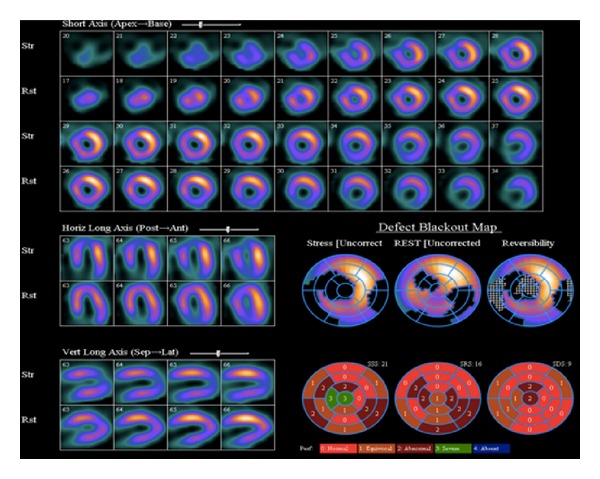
Myocardial perfusion imaging demonstrating a moderate size area of ischemia in the mid to apical anteroseptal, apical, and periapical segments.
